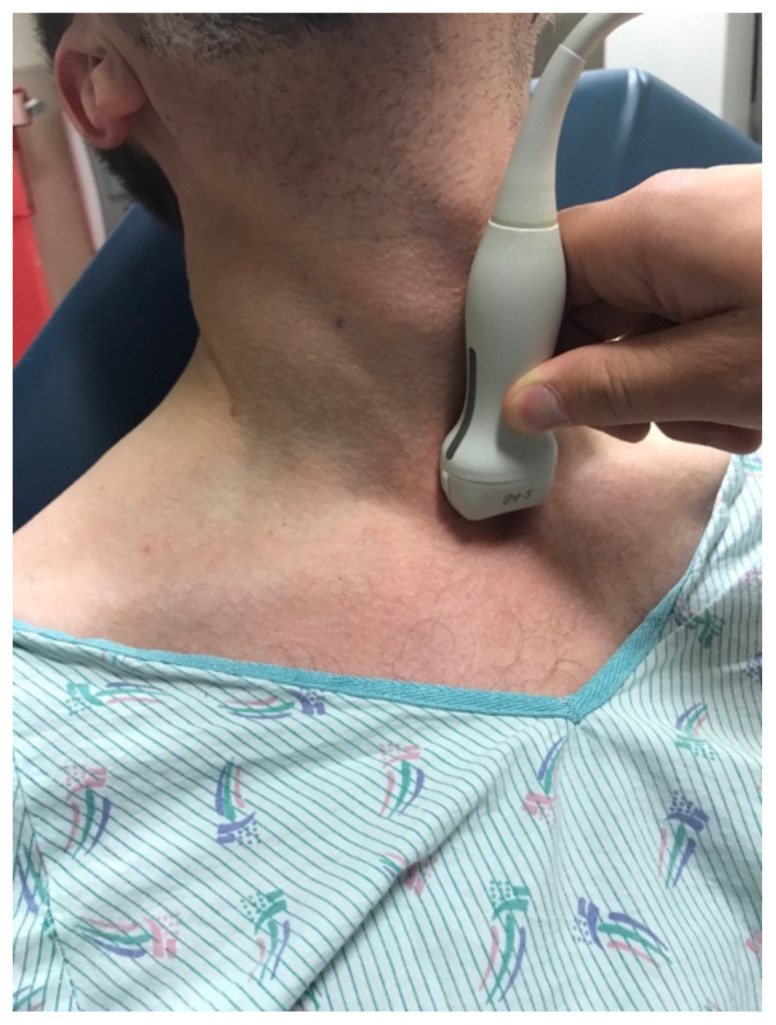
Parasternal Long Video: https://youtu.be/Nfbhj8oEaE0Suprasternal Notch View Video: https://youtu.be/fdqjgsWrxjM
